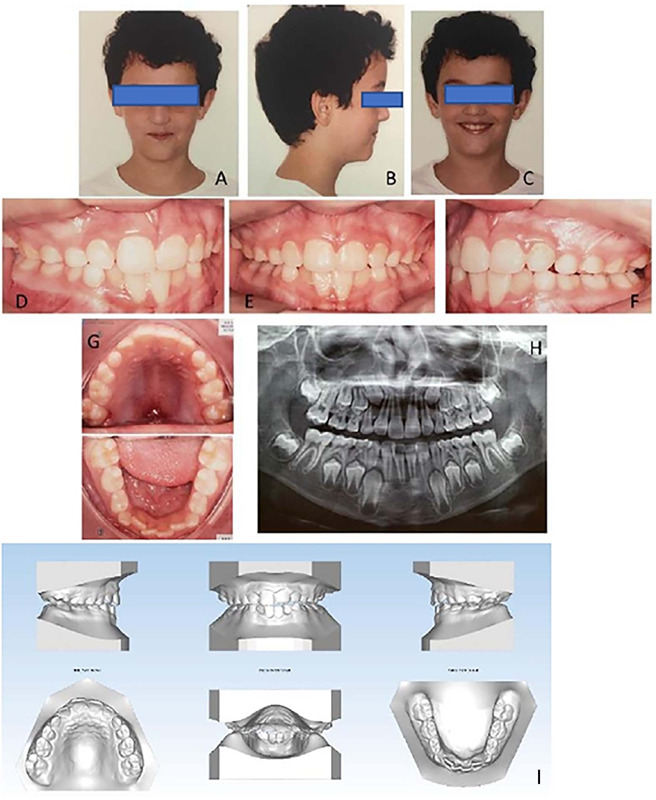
Post-treatment. (A,B,C) extraoral photographs; (D,E,F,G) intraoral photographs; (H) orthopantomograph; (I) intraoral models.
